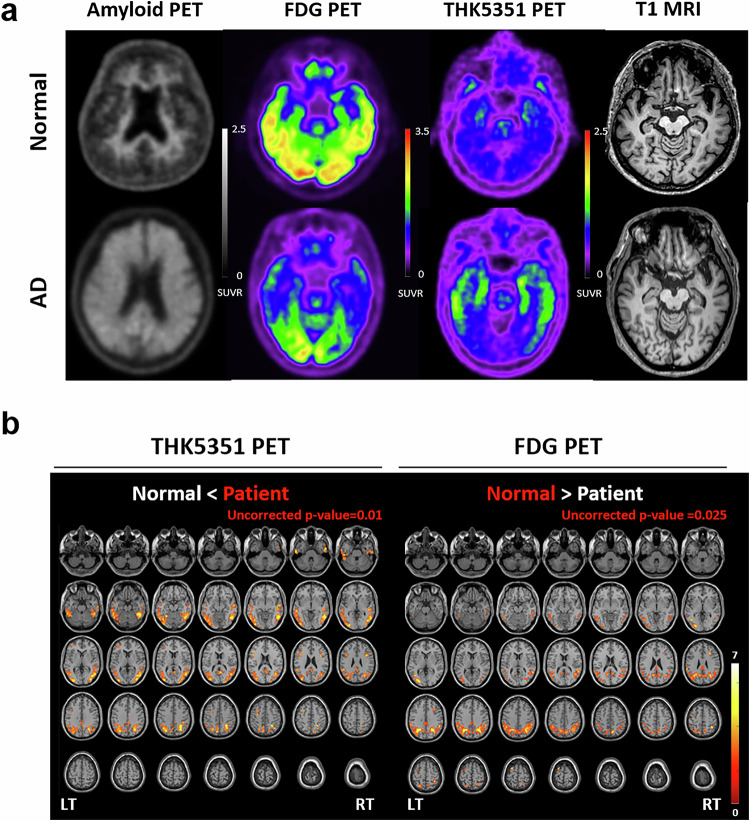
THK5351 uptake in the brain of patients with Alzheimer’s disease. a Amyloid, FDG and THK5351 positron-emission tomography (PET) images in the brain from both healthy control subjects and patients with Alzheimer’s disease (AD). Right: MRI in human brain with AD. b Unique imaging patterns obtained by contrasting normal control individuals with those diagnosed with AD.
